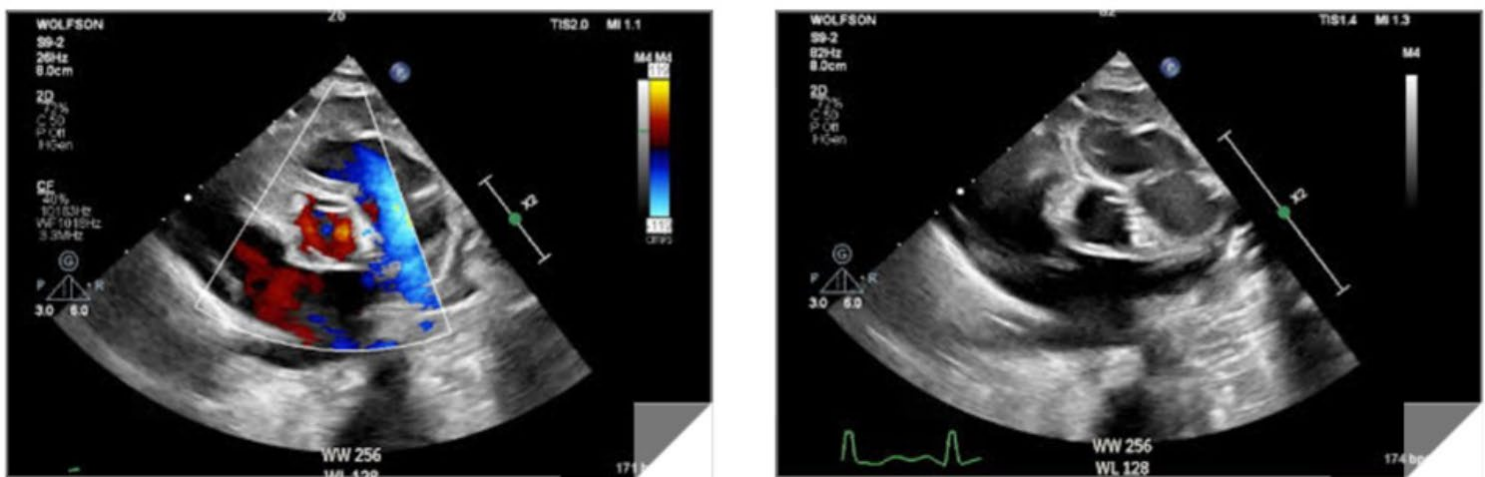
Coronary echocardiographic imaging prior to surgery
